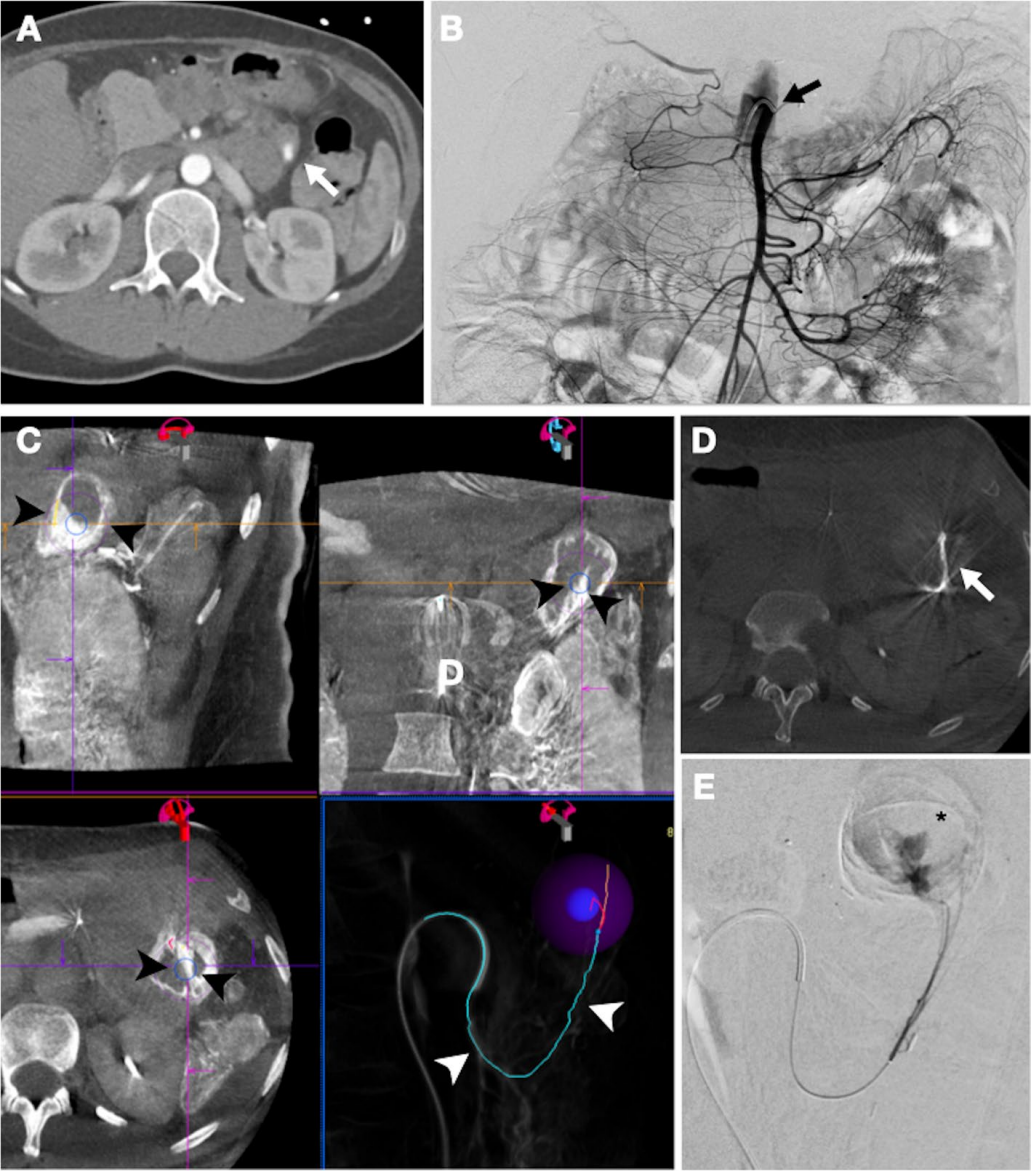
Empiric TAE approach in patient 2 A Pre-procedural arterial-phase CTA displays a contrast extravasation proximally in the Roux-en Y jejunal loop (white arrow). B No bleeding focus on subsequent DSA with contrast injection in the superior mesenteric artery (black arrow). C Superior mesenteric CBCT MPR images in the 4D workplace. Here, using prior CTA bleeding focus, mucosal enhancement in the suspected culprit jejunal bowel segment was highlighted as ROI (black arrowhead) for automatic vessel detection. The resulting 3D vessel segmentation (white arrowhead) was used for catheter navigation. D A CBCT acquisition with super-selective contrast injection in the engaged artery confirmed correct targeting trough the mucosal enhancement (white arrow) overlapping the previously decided ROI (intended bleeding site). E Contrast injection after selecting the targeted artery eventually showed an extravasation (black asterisk)
